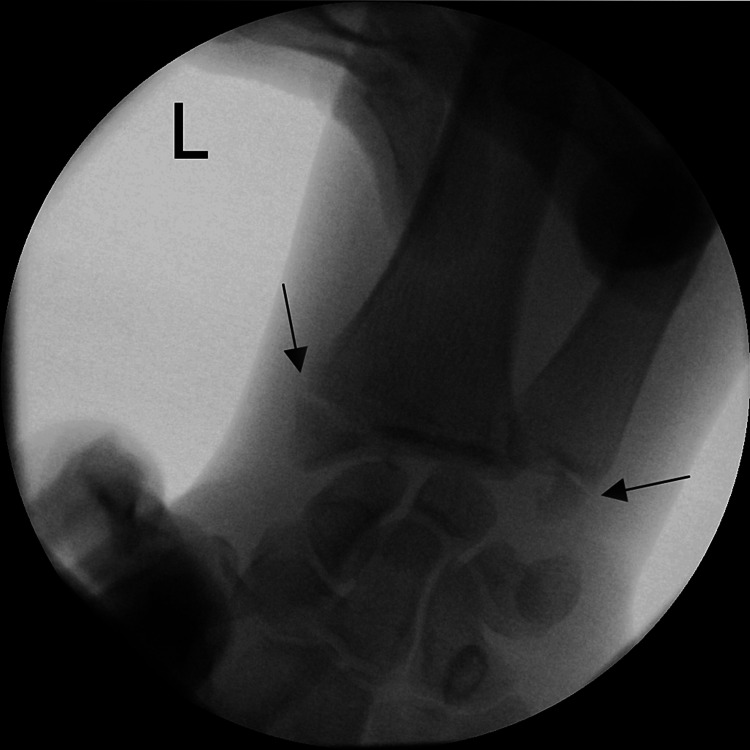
Perioperative X-rays of the wrist, posterior-anterior view Visible fracture of both radial and ulnar styloid processes, but radiocarpal dislocation is not clearly visible in this view.
